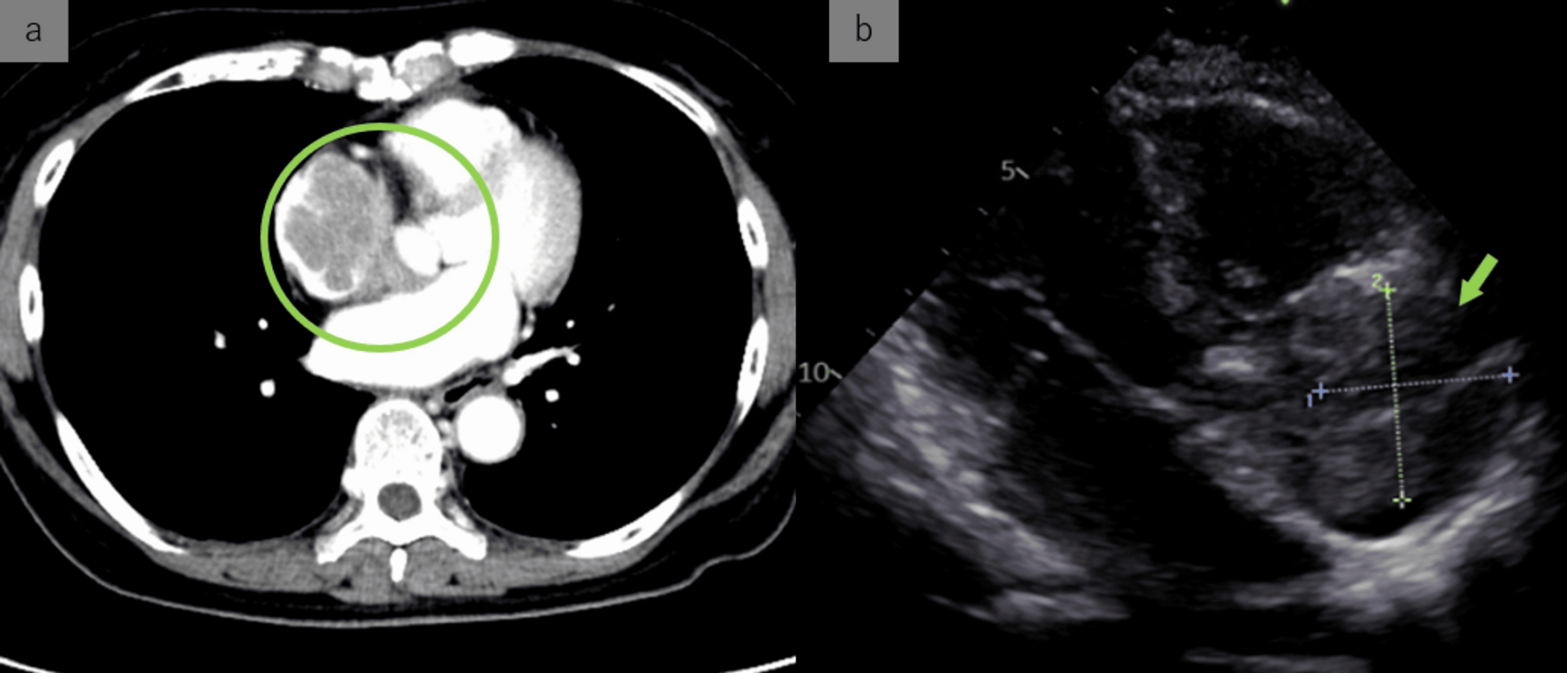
CT scan and echocardiography (a) A routine CT scan during continued pembrolizumab, revealed a contrast defect due to a mass occupying the right atrium. (b) Echocardiography showed the mass occupying the right atrium to be 40 x 33 mm in size.
